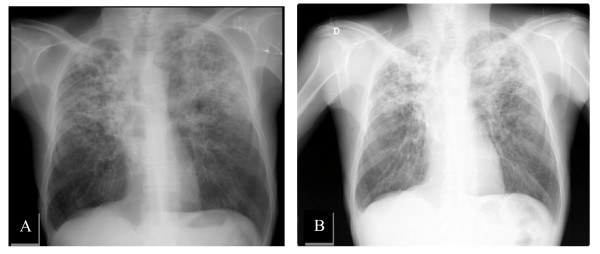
Chest X-ray on admission (A). Chest X-ray 8 months later, showing exsudative lesions on both upper lobes (B).
